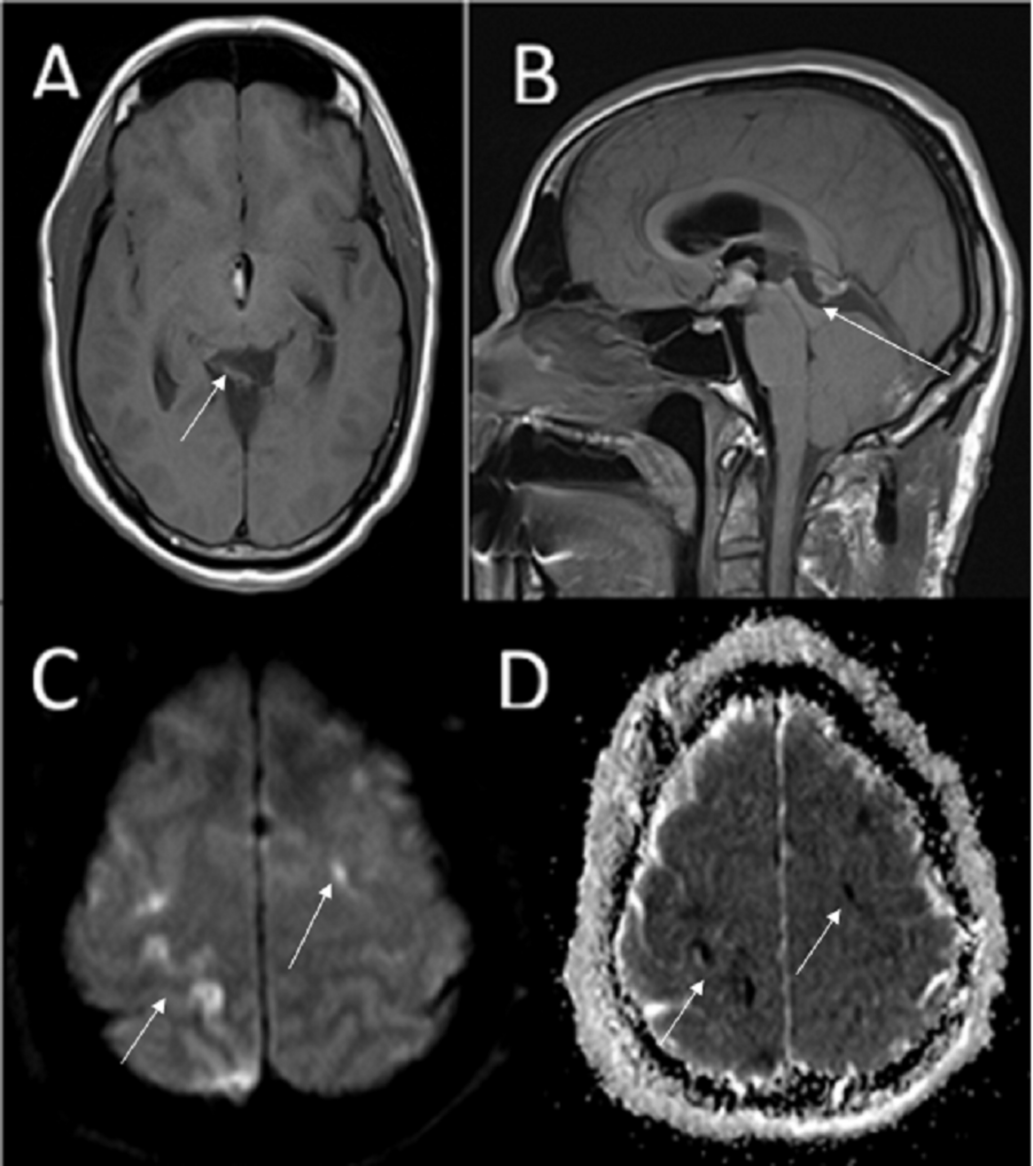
Post-operative magnetic resonance imaging (MRI). (A) Axial T1-weighted image and (B) sagittal T1-weighted image showing resection of the pineal lesion. (C) Diffusion-weighted imaging showing areas of restricted diffusion in the right and left frontal lobes and (D) the corresponding apparent diffusion coefficient (ADC) map confirming this finding. We were unable to obtain contrast-enhanced imaging secondary to patient condition.
